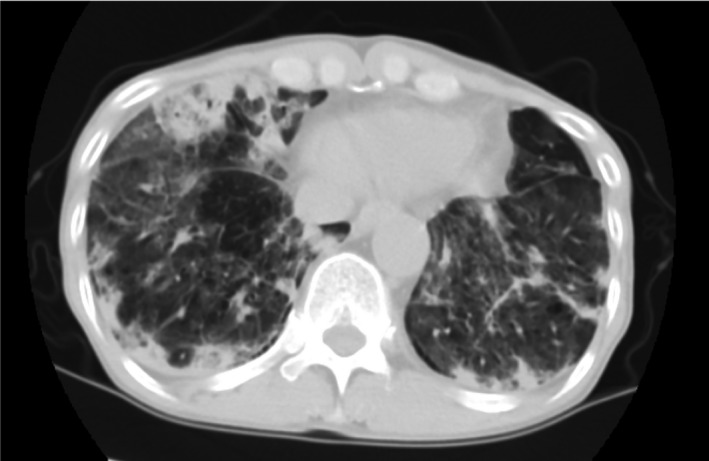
Chest computed tomography scan obtained at admission, showing consolidation and ground‐glass opacity in both the lower lung lobes
